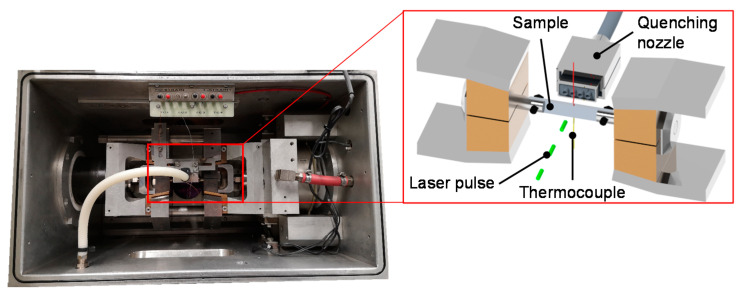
Experimental setup for the evaluation of the microstructural evolution during hot stamping employing a laser-ultrasound sensor.
